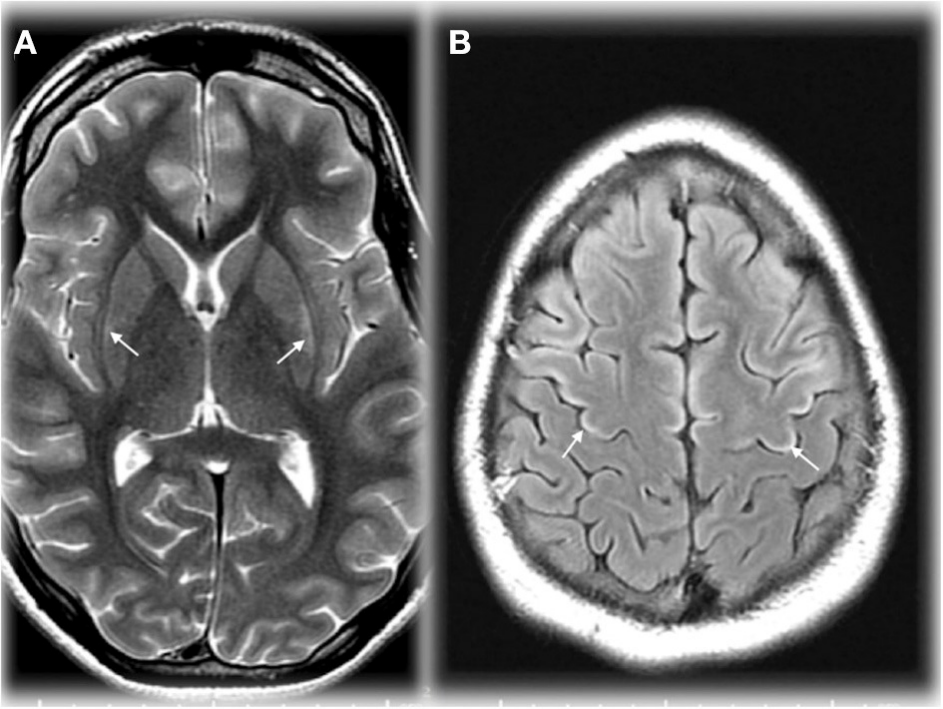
Axial T2 weighted image (TR/TE ms, 4,228/98) through the basal ganglia (A) demonstrating maximal changes in signal in the lateral putamen in an adolescent in OTCD after HA. Axial T2 FLAIR image (IT/TR/TE ms, 2,250/10,000/144) at the cerebral vertex (B) showing cortical hyperintensity in parts of the frontal lobes (motor strip, arrows). Reprinted from, with permission from IOS Press. Sen et al. (62).
